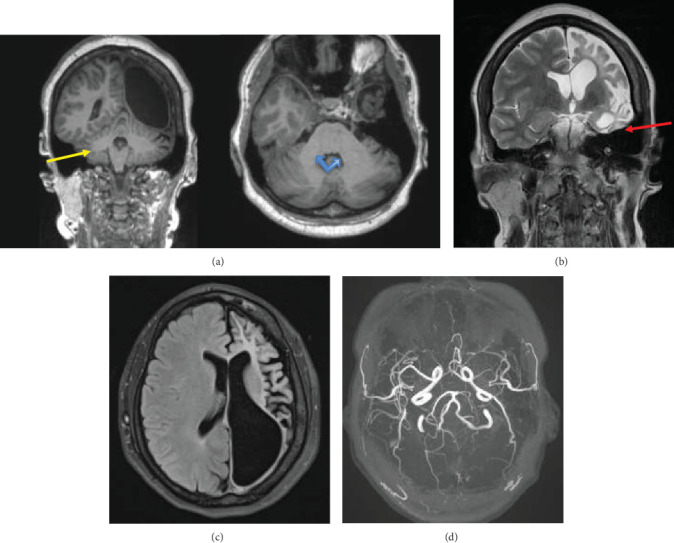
T1-weighted coronal and axial images show atrophy of the right cerebellar hemisphere compared to the left (yellow arrow [a]) with a smaller right middle cerebellar peduncle compared to the left (blue arrow [a]). The coronal T2-weighted image at the level of the petrous apex shows elevation of the left petrous ridge (red arrow [b]). The T2 FLAIR axial image shows no evidence of abnormal signal intensity in the atrophic left cerebral hemisphere (c). The noncontrast 3D-TOF MRA MIP image shows normal cerebral vessels (d).
